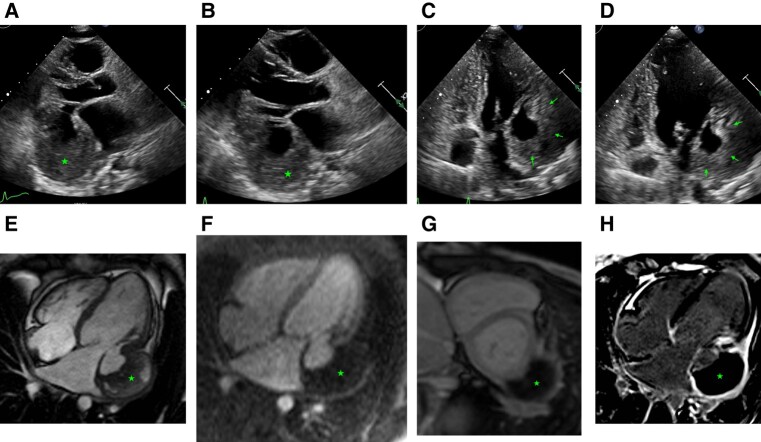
Transthoracic echocardiogram parasternal long-axis view in systole (*A*) and diastole (*B*) revealed a 3.8 × 6.8 cm thickening in the lateral wall of the left ventricle concerning for infiltrative process vs. tumour, indicated by the star. Apical four-chamber view demonstrates this, in systole (*C*) and diastole (*D*), indicated by the arrow. Cardiac magnetic resonance imaging was done on a Siemens Aera 1.5T scanner. Cine sequences were done using steady-state free precession sequences with 0.6 mm slice thickness and 0.4 mm gap. Delayed enhancement sequences were done using high TI imaging with a TI of 600 ms for thrombus evaluation and low TI imaging with a TI of 320 ms, adjusted to the null time, for delayed enhancement and scar imaging. Four-chamber view cine (*E*) shows large left ventricular basal inferolateral pseudoaneurysm, adjacent to the mitral valve, measuring 3.3 cm at the mouth, 4 cm in depth, and 5.9 at the largest dimension, with dense material filling the pseudoaneurysm, suggestive of thrombus (star). Four-chamber perfusion imaging (*F*) showing normal myocardial perfusion and lack of perfusion of the dense material in the pseudoaneurysm, suggestive of a thrombus (star). Short-axis high TI imaging (*G*), with an inversion time of 600 ms, with dark appearance of the dense material in the pseudoaneurysm, consistent with a thrombus (star). Four-chamber delayed enhancement imaging (*H*) showing inferolateral pseudoaneurysm filled with thrombus (star), covered by overlying pericardium.
